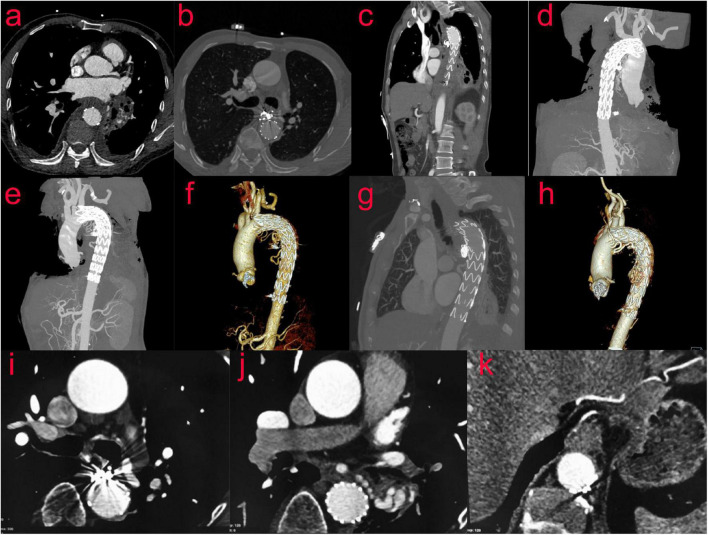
(Case two) CTA of the thoracic aorta was reexamined 10 days after the operation (a–f). After stenting of the aortic arch and descending aorta, the shape and position of the metal stents were evaluated to be good. It adhered to the wall well, and there was no displacement or internal leakage. The mediastinal hematoma was partially absorbed. After 2 months, the reexamination of the thoracic aorta by CTA showed that the mediastinal hematoma was further absorbed, and the stent was in good shape (g,h). Nine months after the operation, the review of CT showed that the mediastinal hematoma was almost completely absorbed (i–k).
